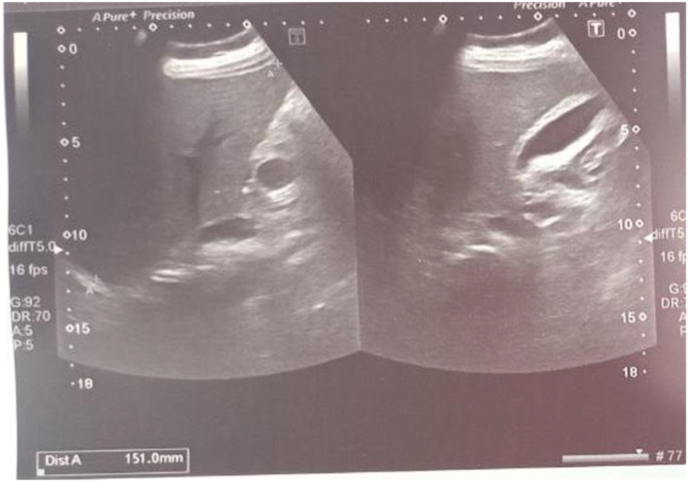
Ultrasonography of abdomen showing normal sized gall bladder with increased wall thickness (5.7mm) suggestive of acute acalculous cholecystitis.
